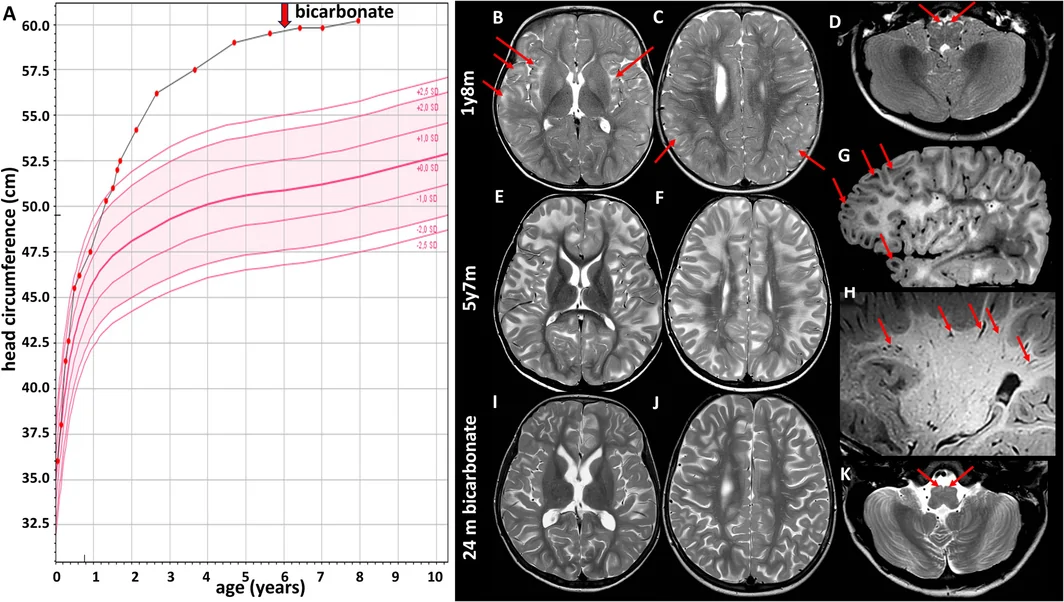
Brain MRI and head circumference in patient 1. (A) Head circumference. The red arrow indicates the start of bicarbonate treatment. (B–D) The first MRI at 1y8m shows minor abnormalities restricted to the directly subcortical cerebral white matter (arrows in B, C) and signal abnormalities in the pyramids and hilum of the inferior olives in the medulla (arrows in D). (E–H) Follow‐up MRI at 5y7m shows an increase in extensiveness and degree of swelling of the cerebral white matter abnormalities, with a decreasing zone of normal periventricular white matter (E, F). Especially, the directly subcortical white matter is rarefied to cystic (arrows in G, also note the anterior temporal cyst). A radiating pattern of enlarged perivascular spaces is seen in the cerebral white matter (arrows in H). (I–K) MRI after 24 months treatment shows a marked decrease in the cerebral white matter edema (I, J), and resolution of the signal abnormalities in the medulla (arrows in K). y, years; m, months.
